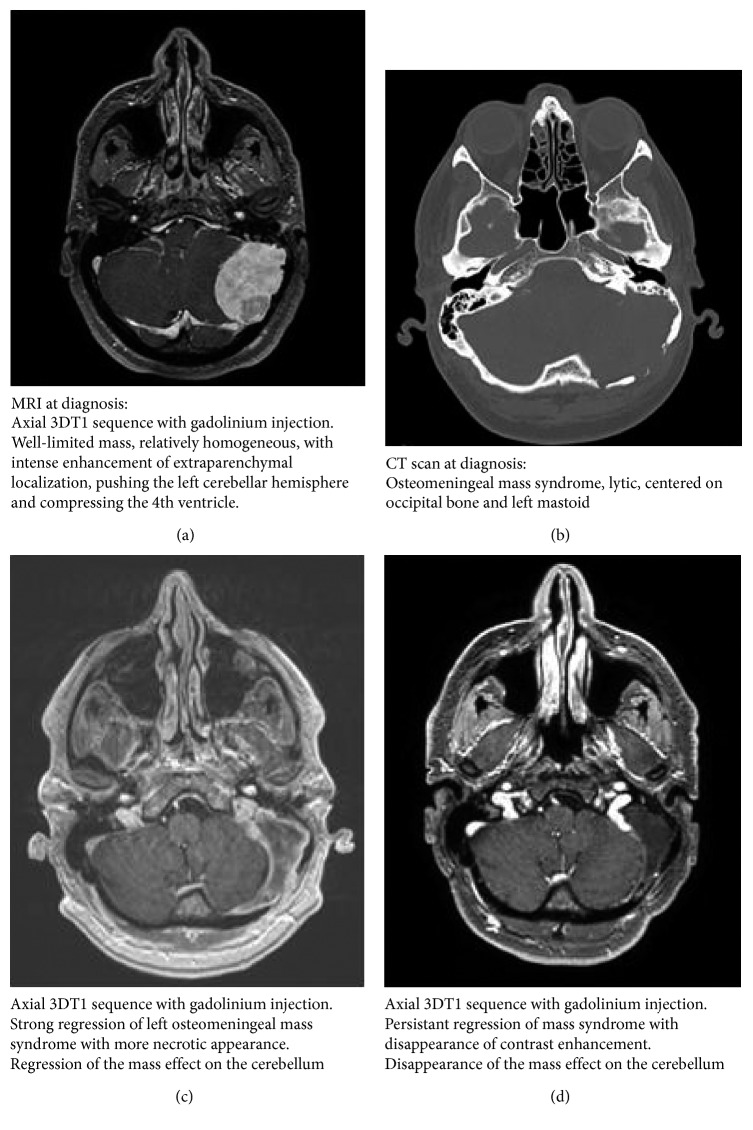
(a) MRI at diagnosis. (b) CT scan at diagnosis. (c) Follow-up MRI after two courses of bortezomib, thalidomide, and dexamethasone. (d) Follow-up MRI after two courses of lenalidomide maintenance.
